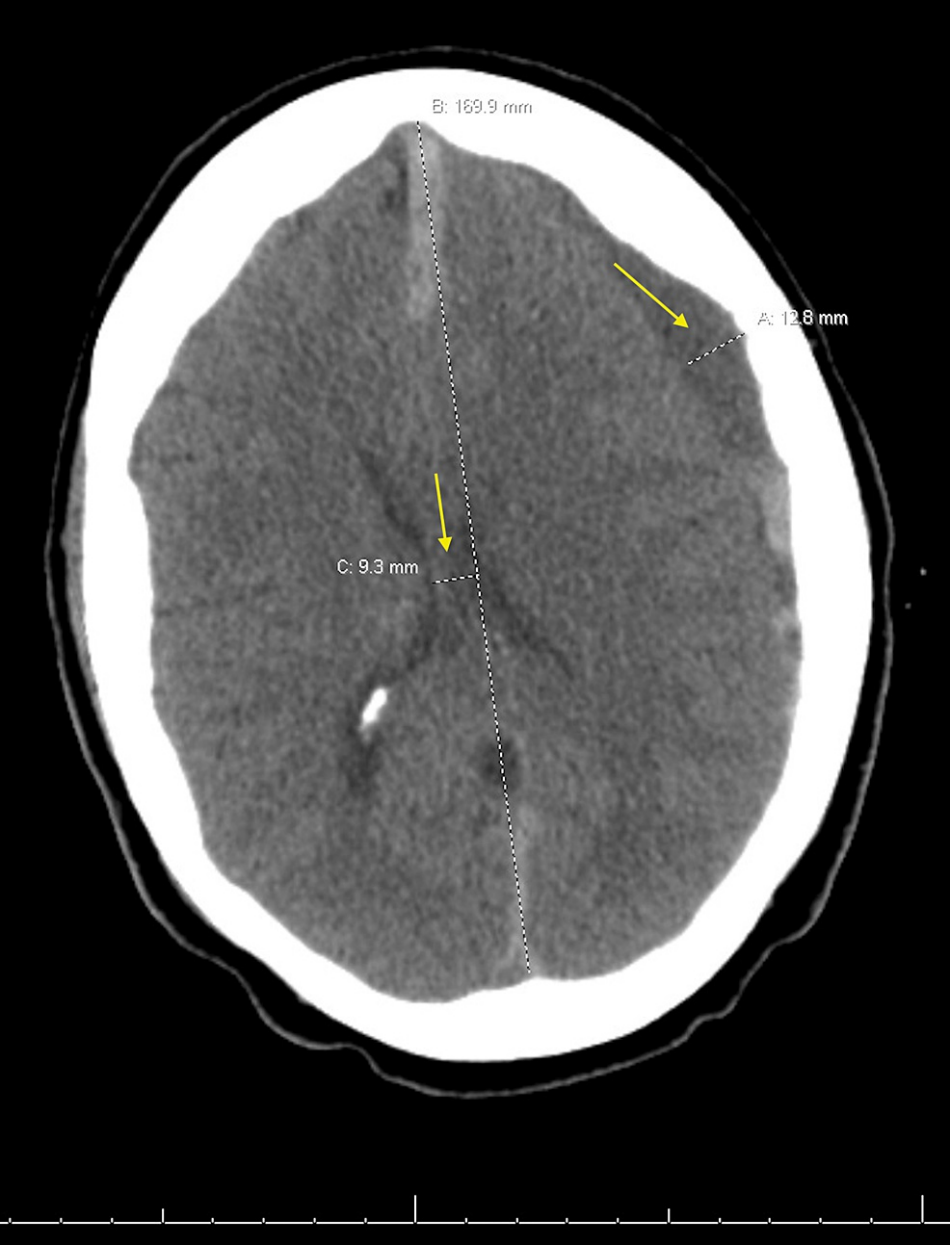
CT scan image of the head CT of the head revealed a 12.8 mm left subdural hematoma with a 9.3 mm midline shift to the right (yellow arrows).
